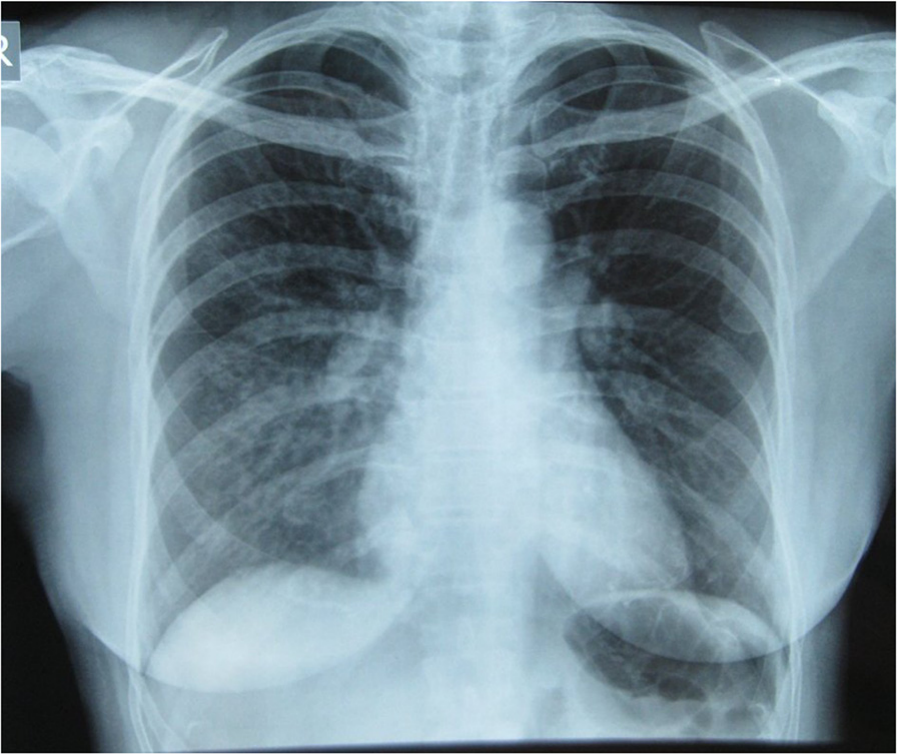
Initial Chest x-ray showing pulmonary infiltrates in the right lung especially in the right mid and lower lung zones indicative of pulmonary edema.
